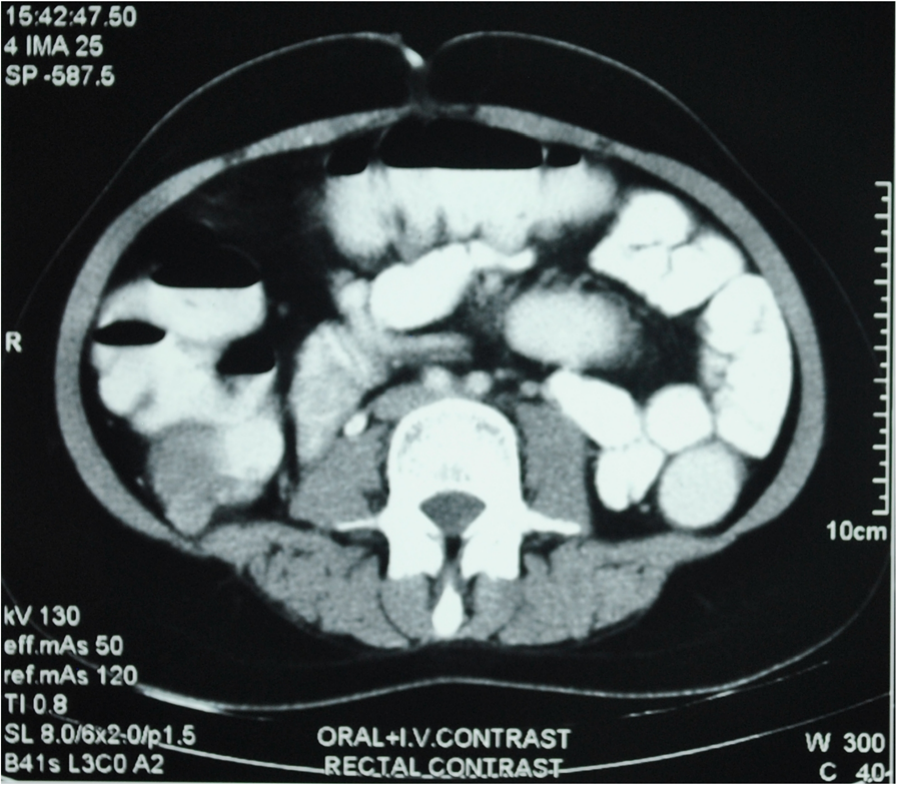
Computed tomography image showing mass at the ileocolic junction; no recurrence is seen in the renal bed
